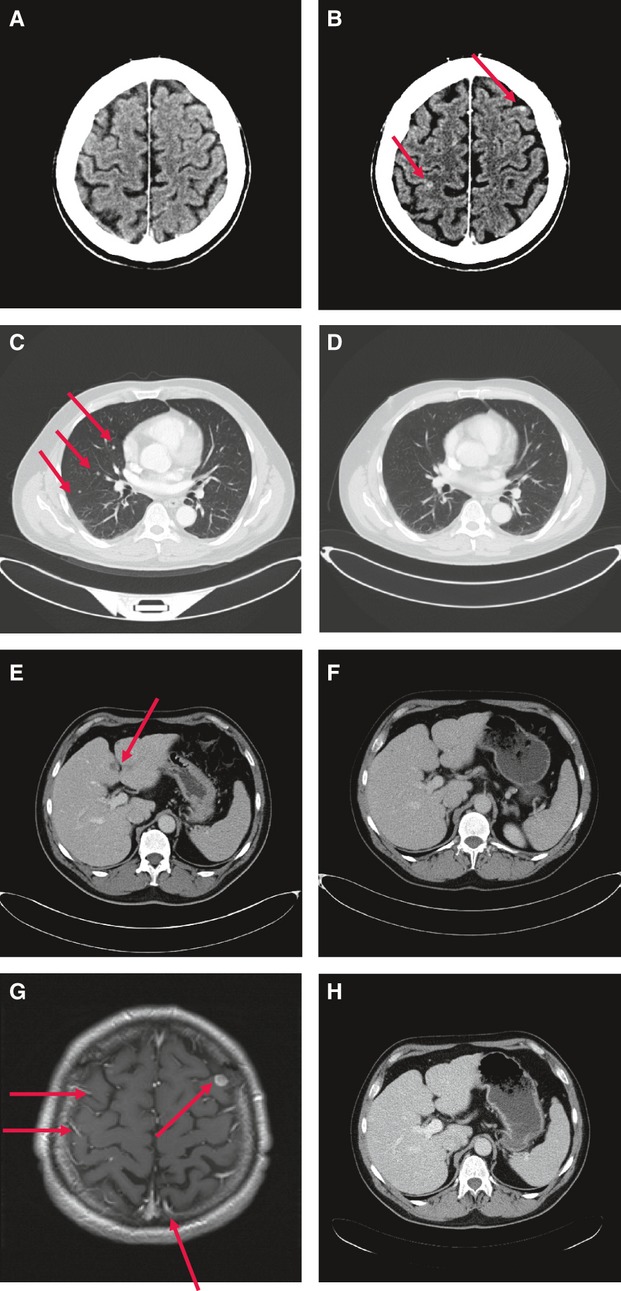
Computed tomography (CT) scans before and after treatment with vemurafenib (A–F) or ipilimumab (G and H) (patient 2). (A) CT scan (June 2010) before treatment: no cerebral metastases. (B) CT scan (August 2011) after treatment: new cerebral metastases. (C) CT scan (June 2010) before treatment: pulmonary metastases. (D) CT scan (August 2011) after treatment: complete remission. (E) CT scan (June 2010) before treatment: liver metastases. (F) CT scan (August 2011) after treatment: complete remission. (G) Magnetic resonance tomography (November 2011) after treatment with ipilimumab: progression of brain metastases with leptomeningeal metastases. (H) CT scan (November 2011) after treatment with ipilimumab: complete remission of liver metastases.
